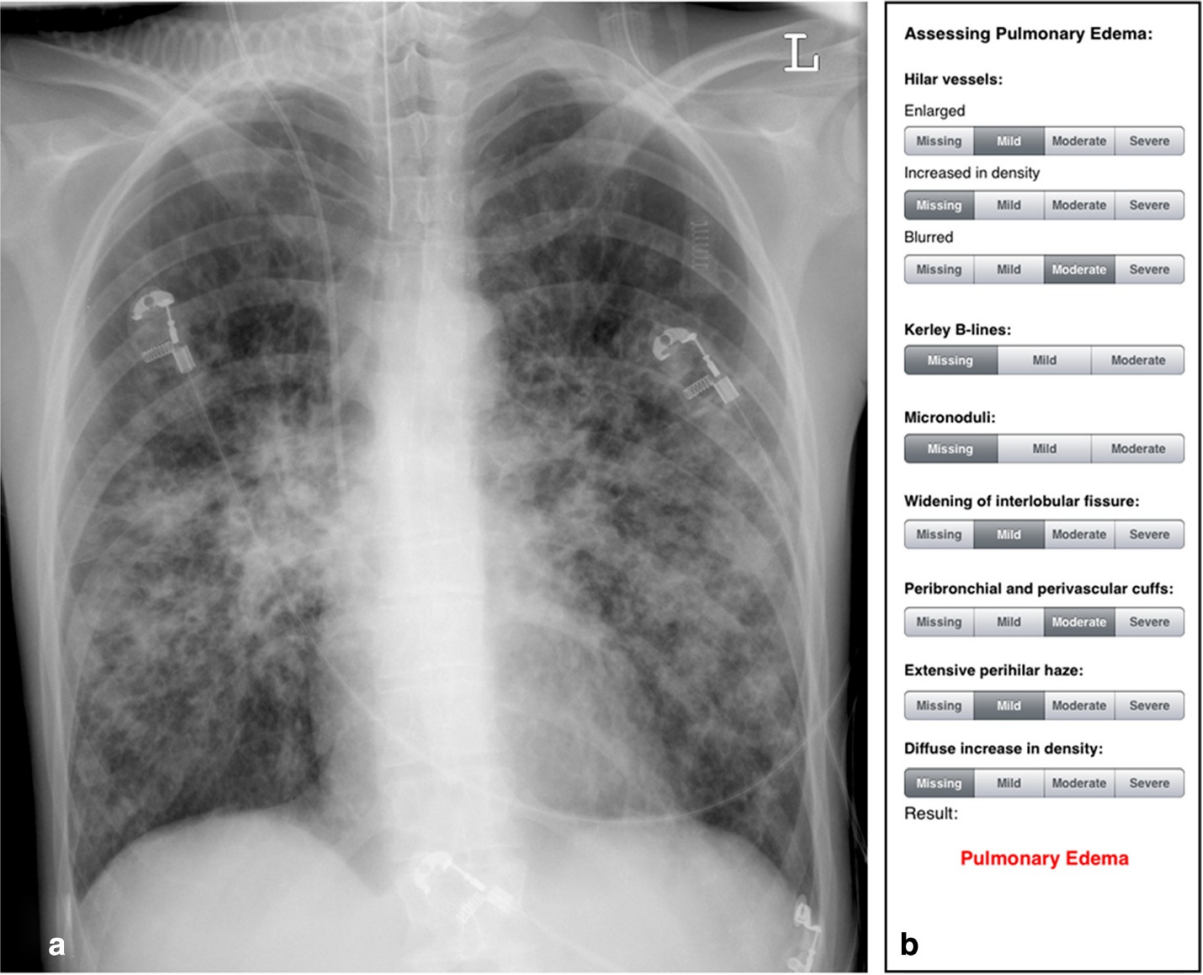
Example bedside chest radiograph with indications of pulmonary edema. a) Pulse contour cardiac output (PiCCO) measurements showed an extravascular lung water (EVLW)-value of 21 mL/kg, indicating pulmonary edema. b) Results of the score-based approach (total score = 24, indicates pulmonary edema).
